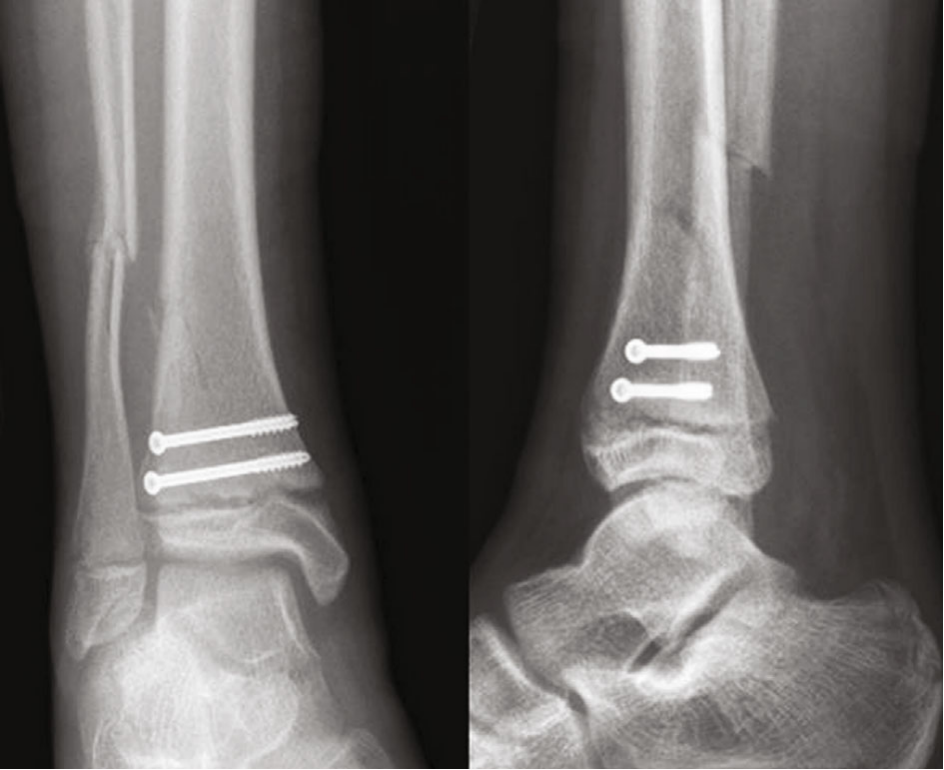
Postoperative radiograph in the anteroposterior and lateral views with fixation by screws.
